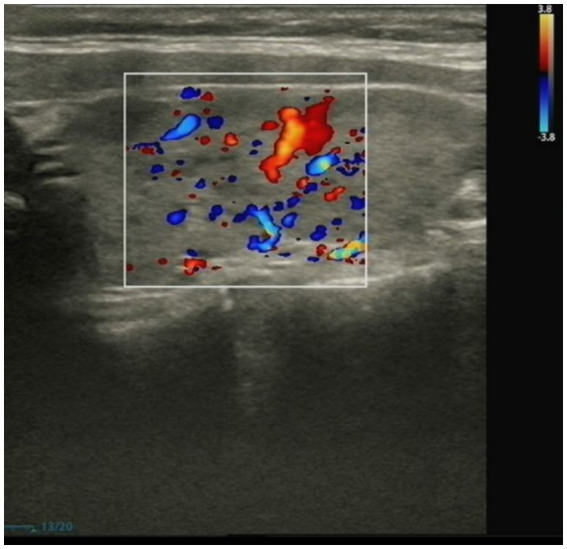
The representative ultrasonographic image of the thyroid of the patient.
